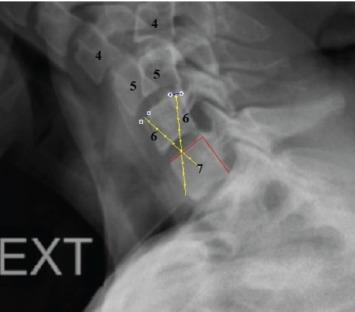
The ICR detection for the C6-C7 segment of the patient in the Fig 1. The ICR was localised at the intersection of the vertices from the midpoint of the two lines. The two lines were formed by the four dots on the anterior and posterior corner of C6 vertebrae in flexion and extension radiographs.
